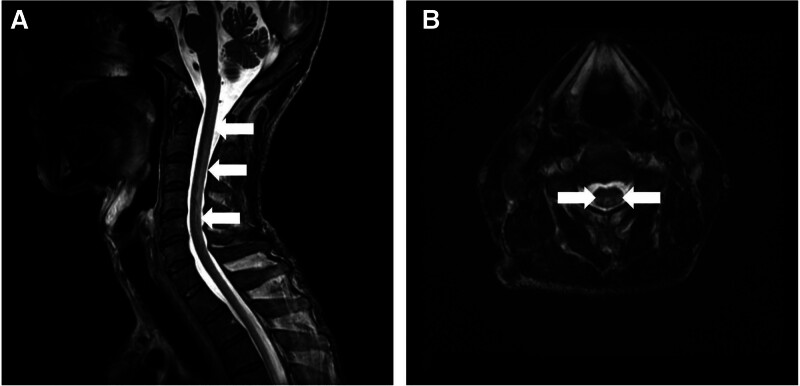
Cervical spine MRI of the patient with SCD. T2-weighted sagittal (A) and axial (B) images show high signal intensity in the dorsal columns (white arrows), consistent with demyelination. MRI = magnetic resonance imaging, SCD = subacute combined degeneration.
